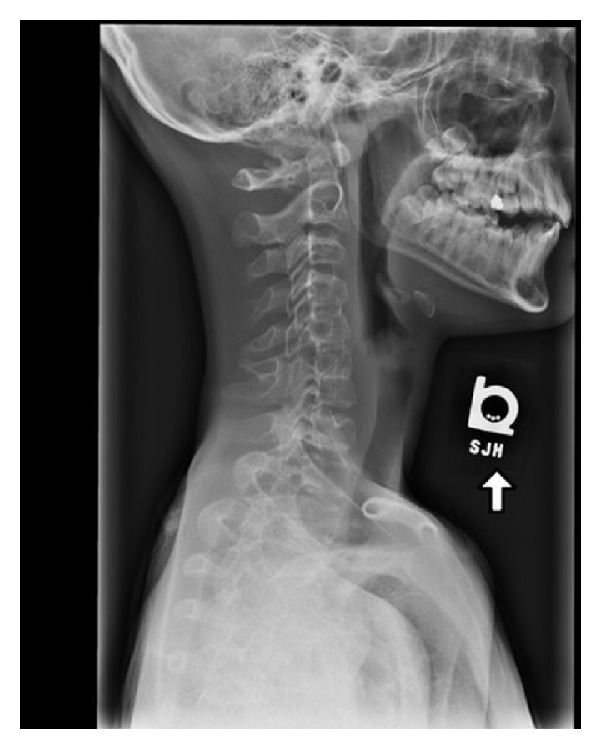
X-ray neck revealing Klippel-Feil anomaly.
